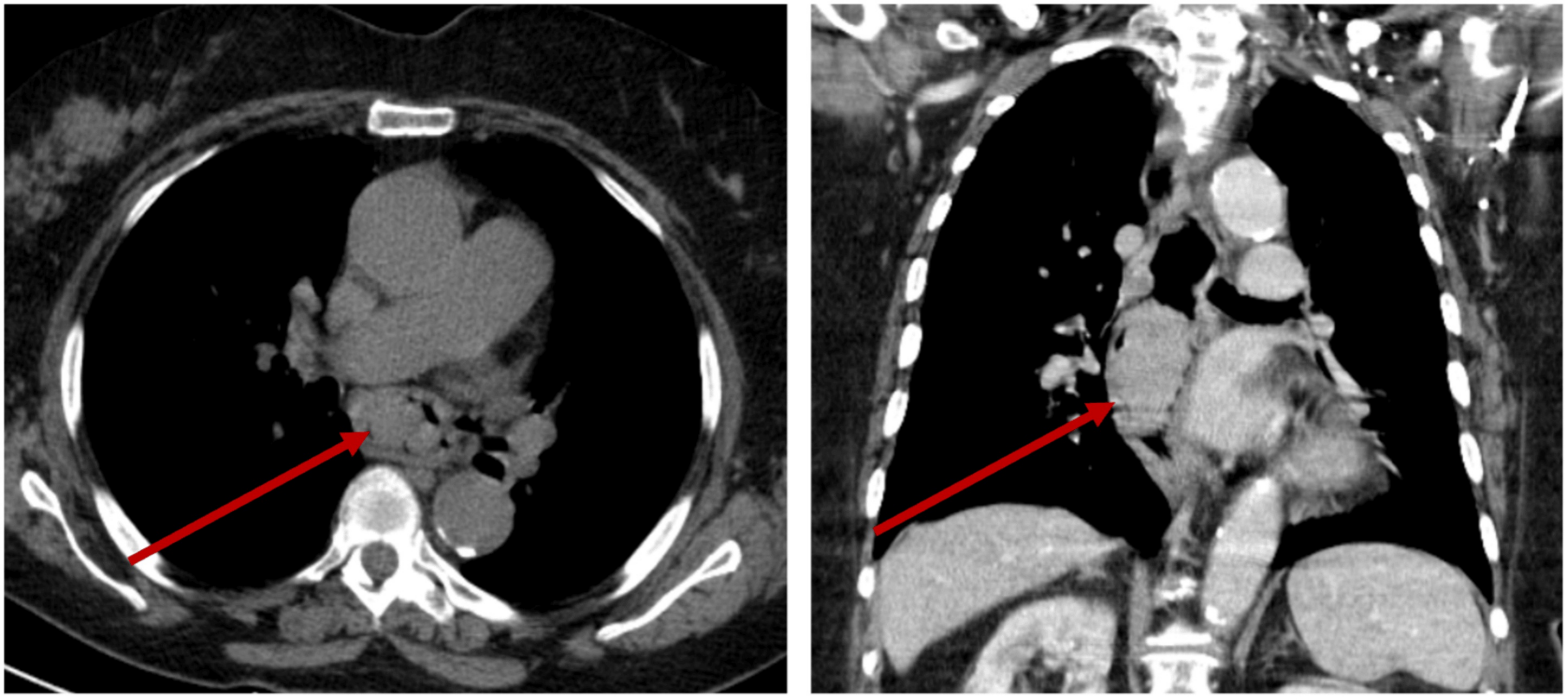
A CT scan showing the exophytic expansive lesion in the middle third of the esophagus, measuring approximately 3.7 x 3 cm axially, with a 5.3 cm craniocaudal dimension, with homogeneous contrast enhancement (red arrow)
